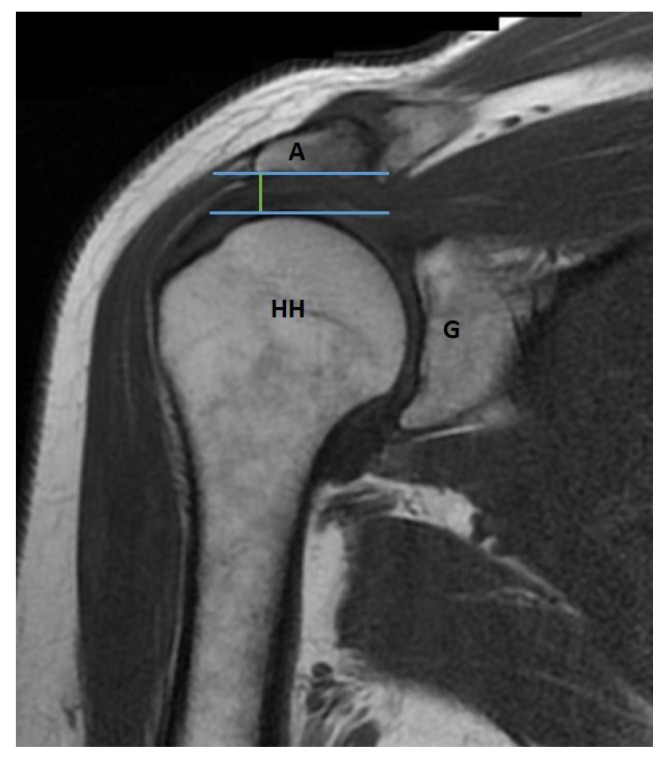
Acromiohumeral distance (AHDsag-green line) on sagittal oblique sections of magnetic resonance imaging scans.
